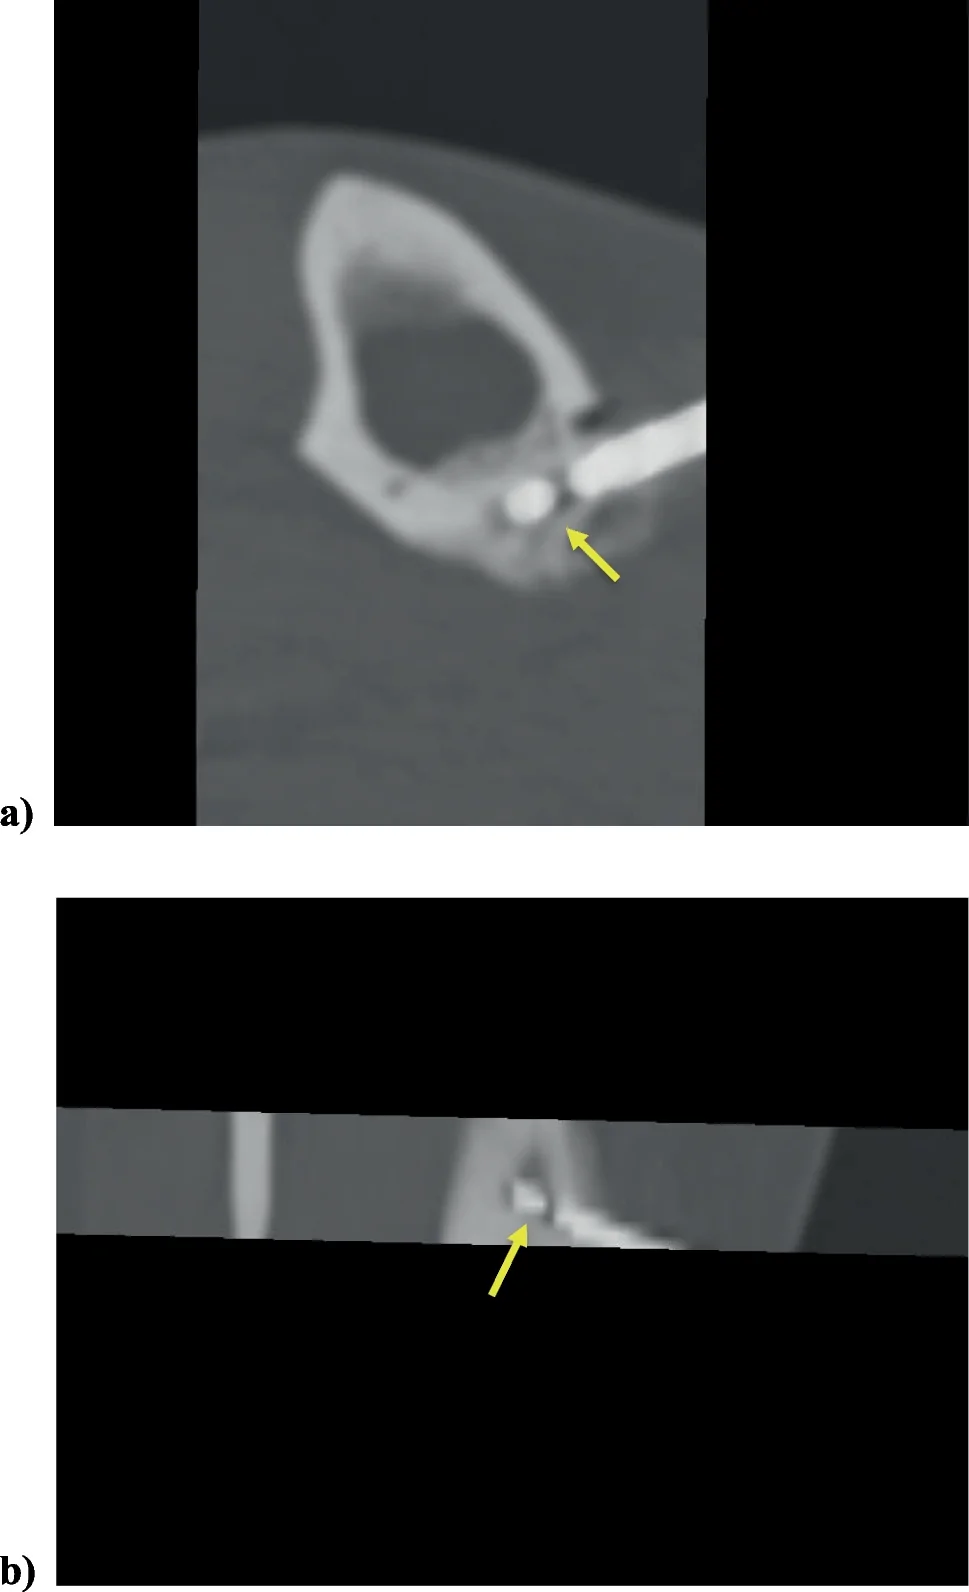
CT-guided RFA of the subperiosteal nidus in a axial and b coronal oblique views. Arrows identify the radiofrequency ablation probe tip within the nidus, which is partially obscured by the probe
